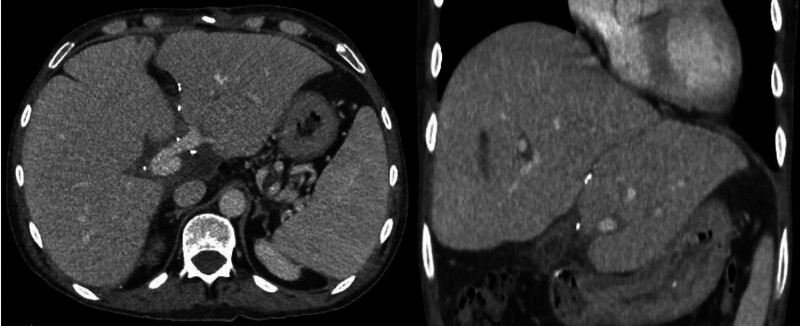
Computed tomography after 1 mo of transplant.
